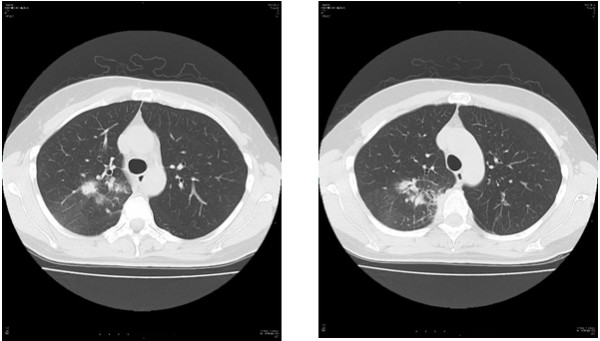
Chest CT view. Chest CT revealed a mass density with 12-, 13- and 16-mm spiculations in the S2 segment of the right upper lobe and irregular thickening of the pleura including the paravertebral region.
